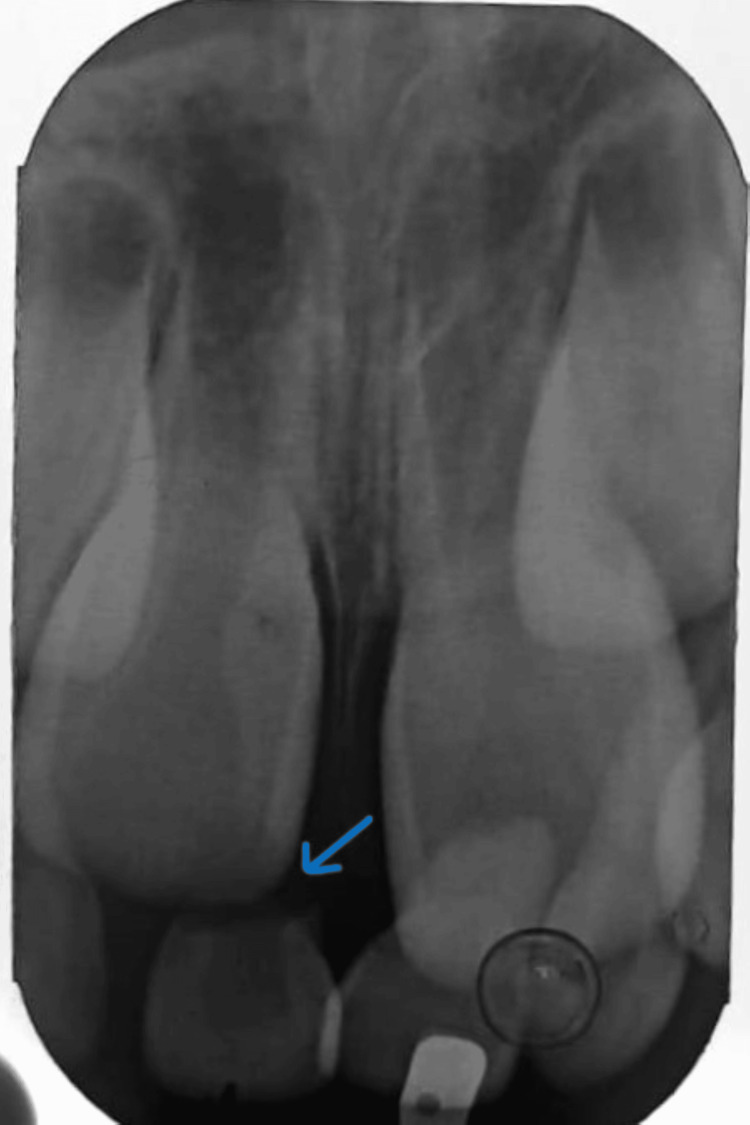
Radiograph of maxillary anterior region showing, primary maxillary right central incisor with root resorption.
